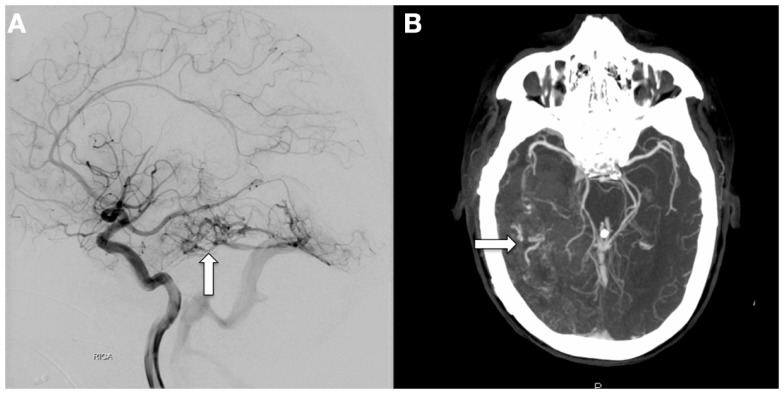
Catheter-based, diagnostic cerebral angiogram (A) and CT-angiogram (B) taken following the CT scans on the left and right of Figure 2, respectively, showing a large tangle of abnormal vessels centered in the right temporal lobe covering an area of at least 7.8 cm in the AP dimension (arrows). The right P2 segment appears to feed a portion of this vascular malformation, and an enlarged vein appears to drain into the basal vein of Rosenthal. These findings are suggestive of AVM.
